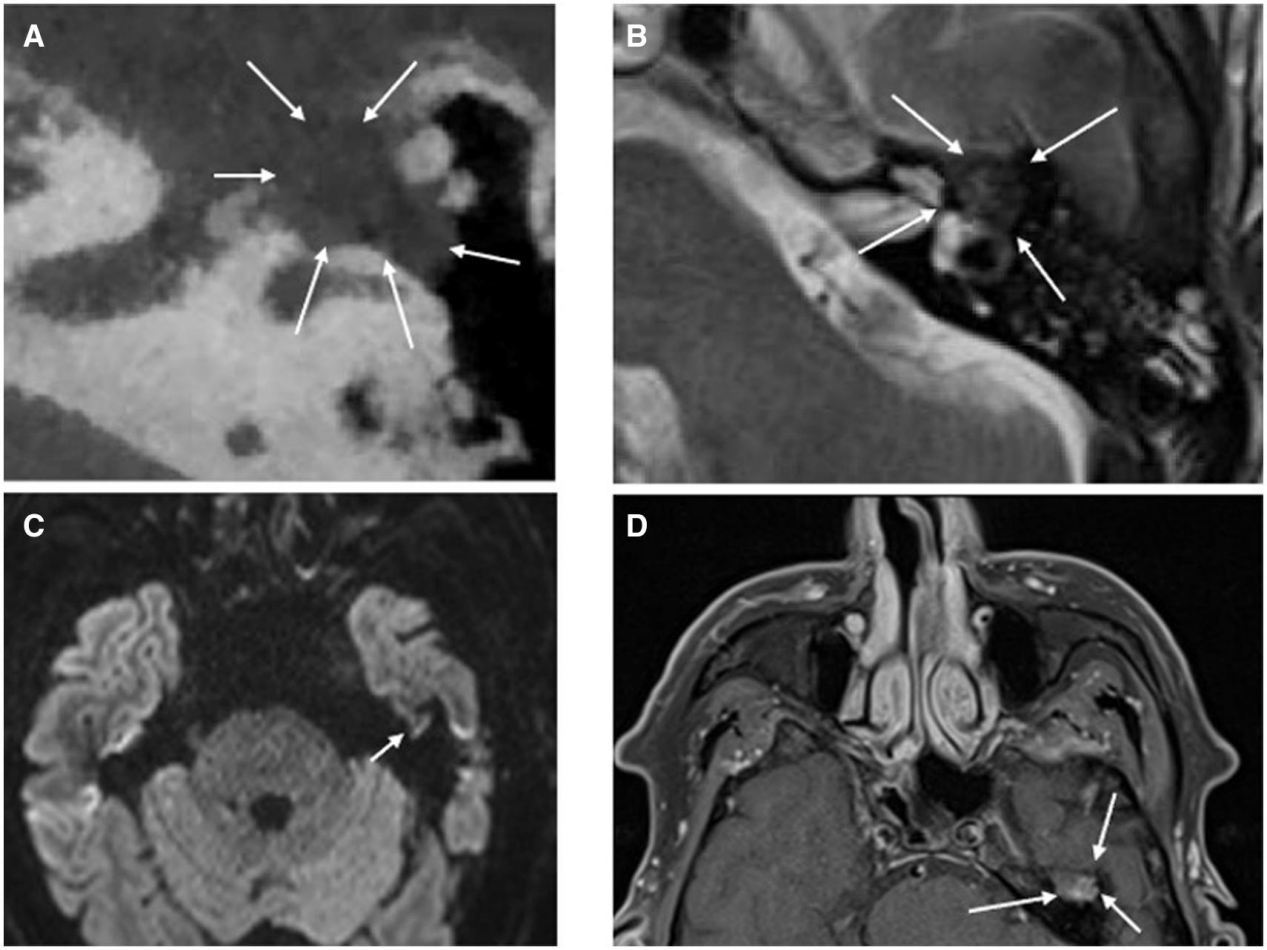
EMP centred on the left geniculate ganglion. (A) Axial non-contrast cone beam CT of the left temporal bone demonstrates an expansile mass (arrows) within the middle ear eroding the otic capsule, geniculate ganglion and tegmen tympani, and laterally displacing the ossicles. (B) Axial T2-weighted MRI shows an intermediate-to-low signal lesion (short white arrow) which restricts on figure (C) and enhances on the (D) fat-saturated post-contrast T1-weighted image (white arrow).
